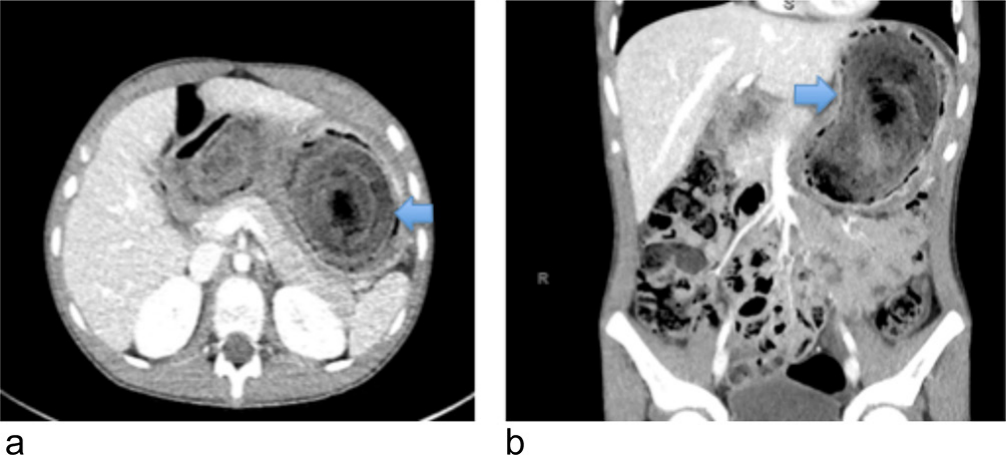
Contrast enhanced axial and coronal CT C/A/P on 1 February 2018: demonstrating a huge oval, heterogeneous, well defined, solid appearing, non-enhancing intraluminal mass, measuring 13 × 6 × 6 cm, in a distended stomach (blue arrows). With no extension of the large mass through the pyloric canal.
